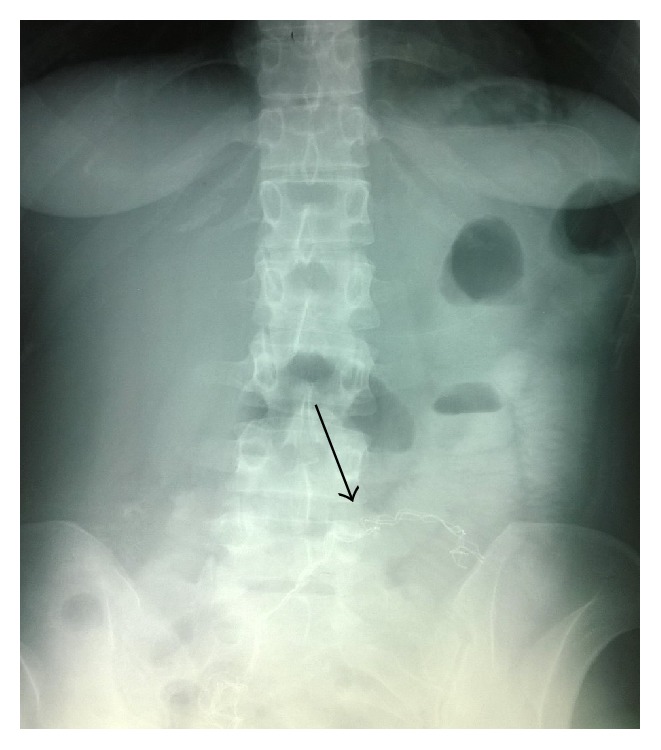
Plain abdominal radiograph revealing a gauze in abdominal cavity.
